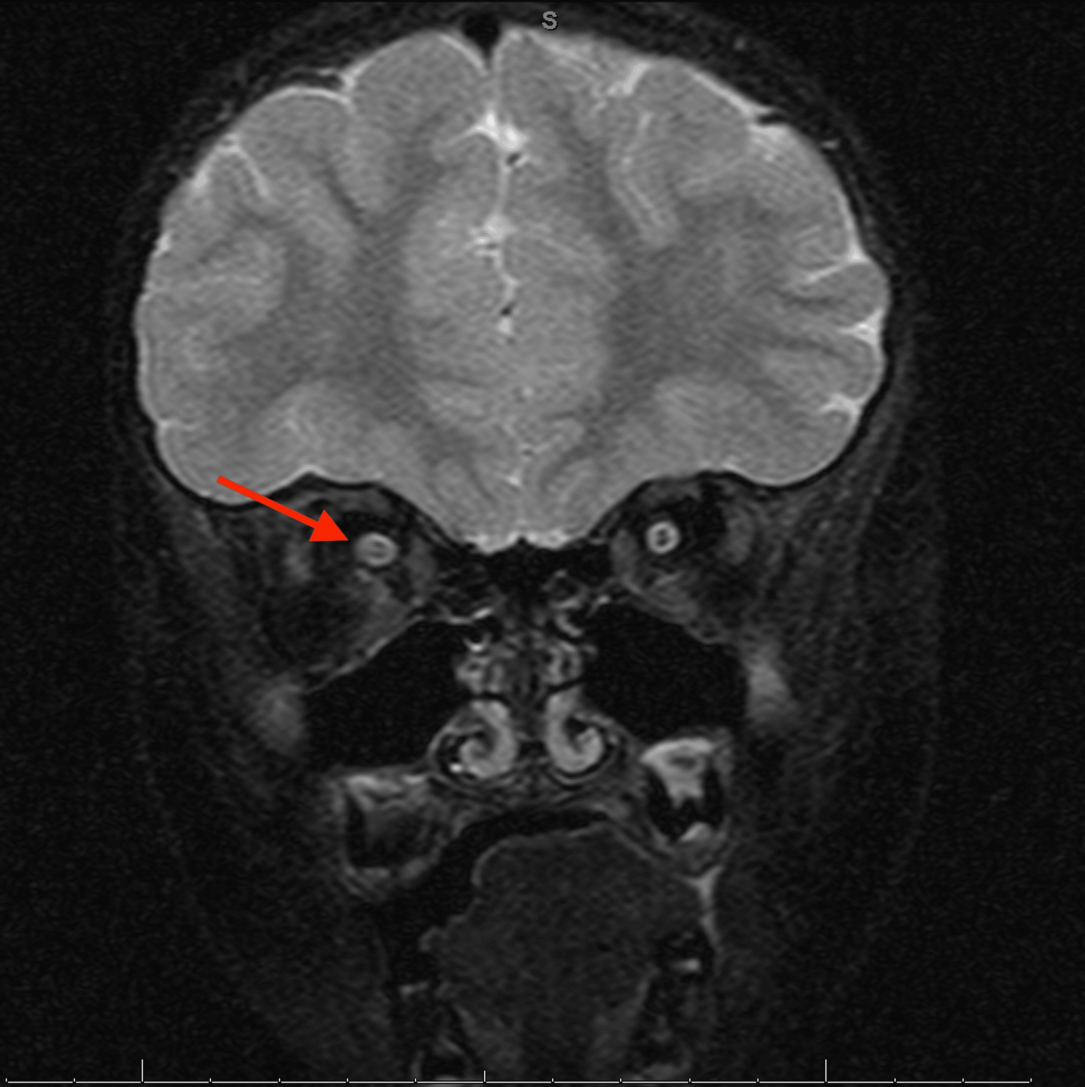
Coronal T2-weighted MRI of the orbits demonstrates questionable increased T2 signal involving the intraorbital segment of the right optic nerve (red arrow), without definite enlargement or abnormal enhancement.
